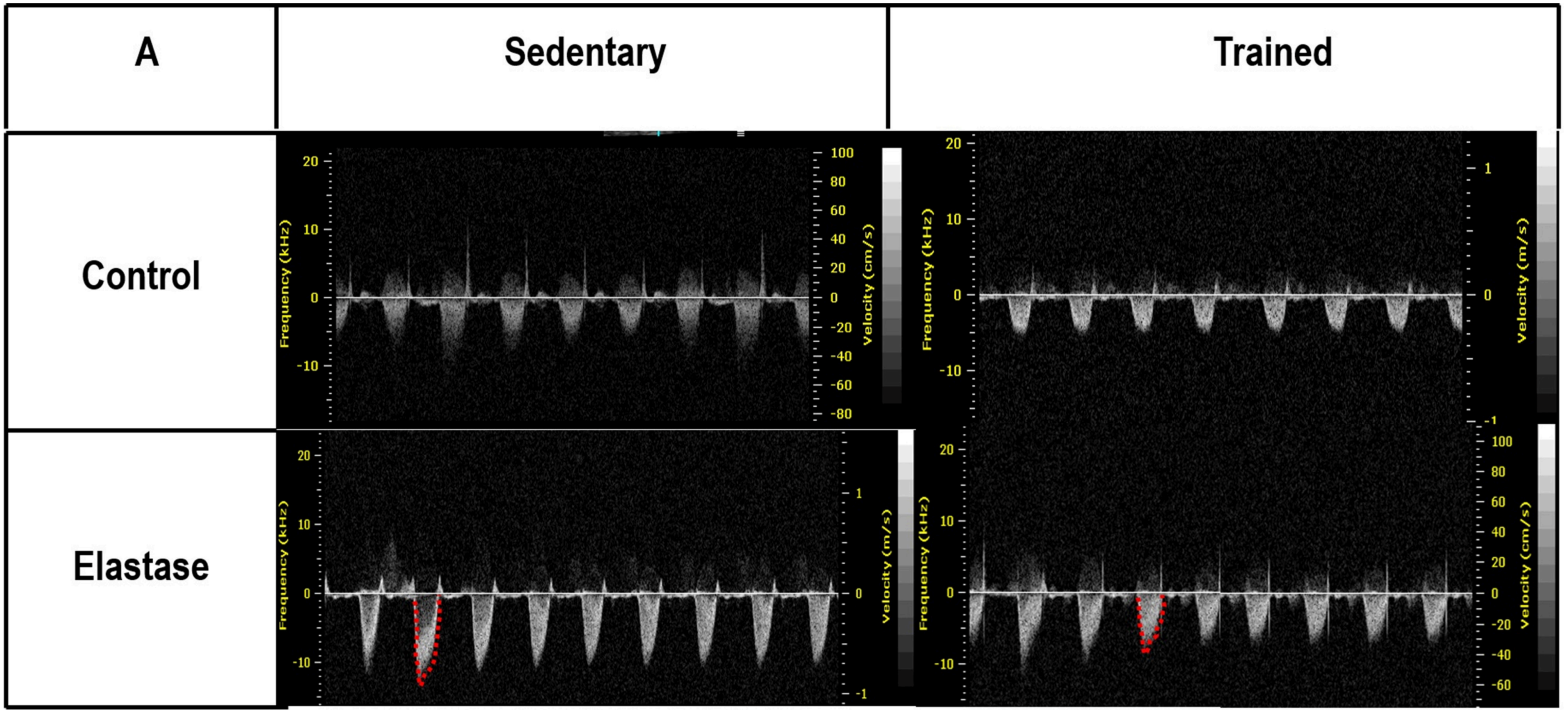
Echocardiographic parameters. Pulmonary artery Doppler flow pattern. C, control. ELA, elastase-induced emphysema. S, sedentary. T, trained.
